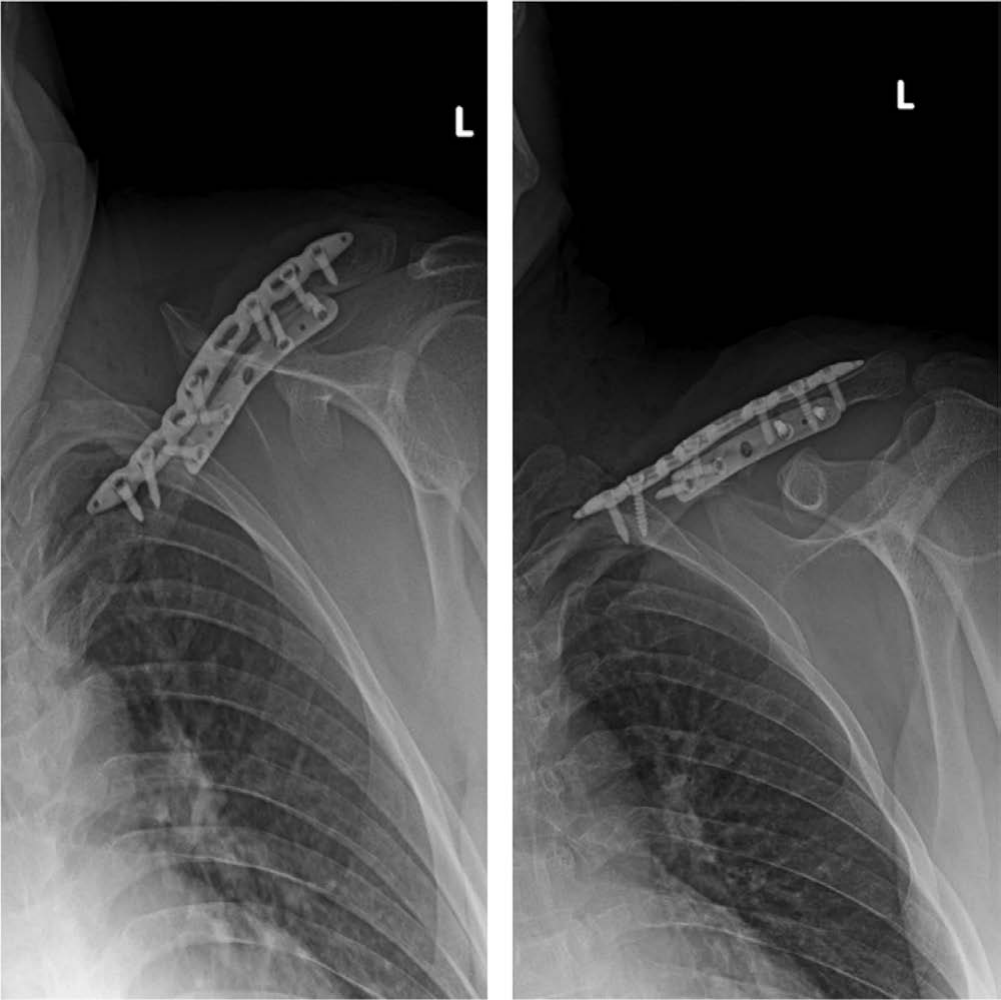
Postoperative X-rays of the patient with double-plate (30 years old, male patient).
